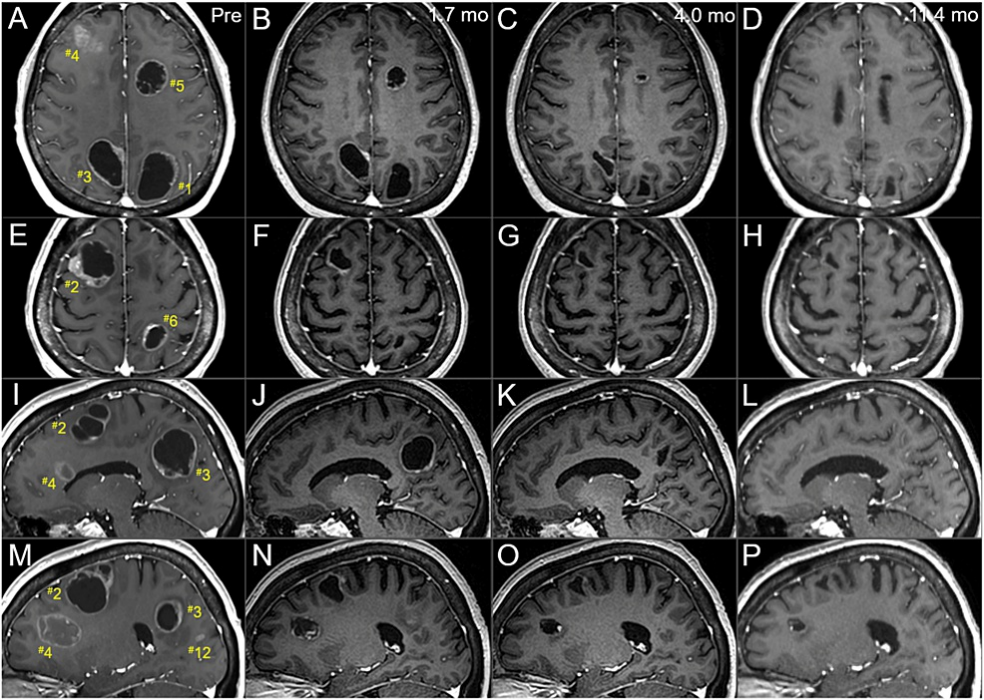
Serial magnetic resonance images before and after the radiotherapy, mainly focusing on the right hemisphere lesions. The images show CE T1-WIs (A-P); axial images (A-H); sagittal images in the right hemispheres (I-P); before the SRS boost (at 8 fr of the WBRT) (A, E, I, M); at 1.7 months after the initiation of WBRT (29 days after the completion of SRS boost) (B, F, J, N); at 4 months after the WBRT initiation (C, G, K, O); and at 11.4 months (D, H, L, P). (A-P) These images are shown at the same magnification and coordinates under co-registration and fusion based on the pre-SRS images. All the seven lesions remarkably shrunk at 1.7 months and further regressed after that at 11.4 months, with the solid components almost disappearing. CE: contrast-enhanced; WIs: weighted images; SRS: stereotactic radiosurgery; fr: fractions; WBRT: whole-brain radiotherapy
